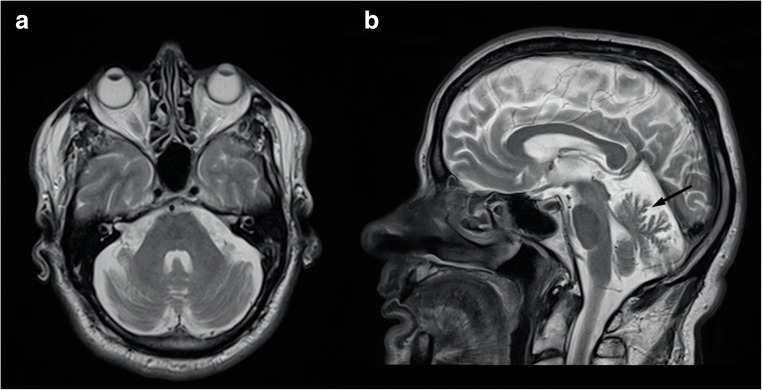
Imaging finding in a 69-year-old male SPG7 patient. Brain MRI axial (a) and sagittal T2- (b) weighted images show the presence of a mild global cerebellar atrophy, with particular involvement of the vermis (black arrow)
